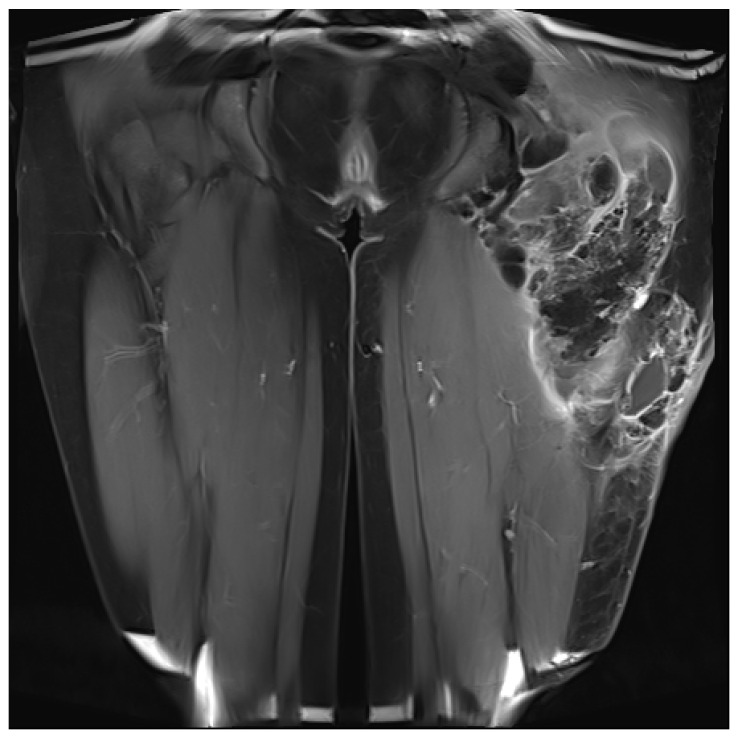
Calcinosis tumor with solid and liquid portions, MRI T2 Sequence. ©UKGM Giessen.
